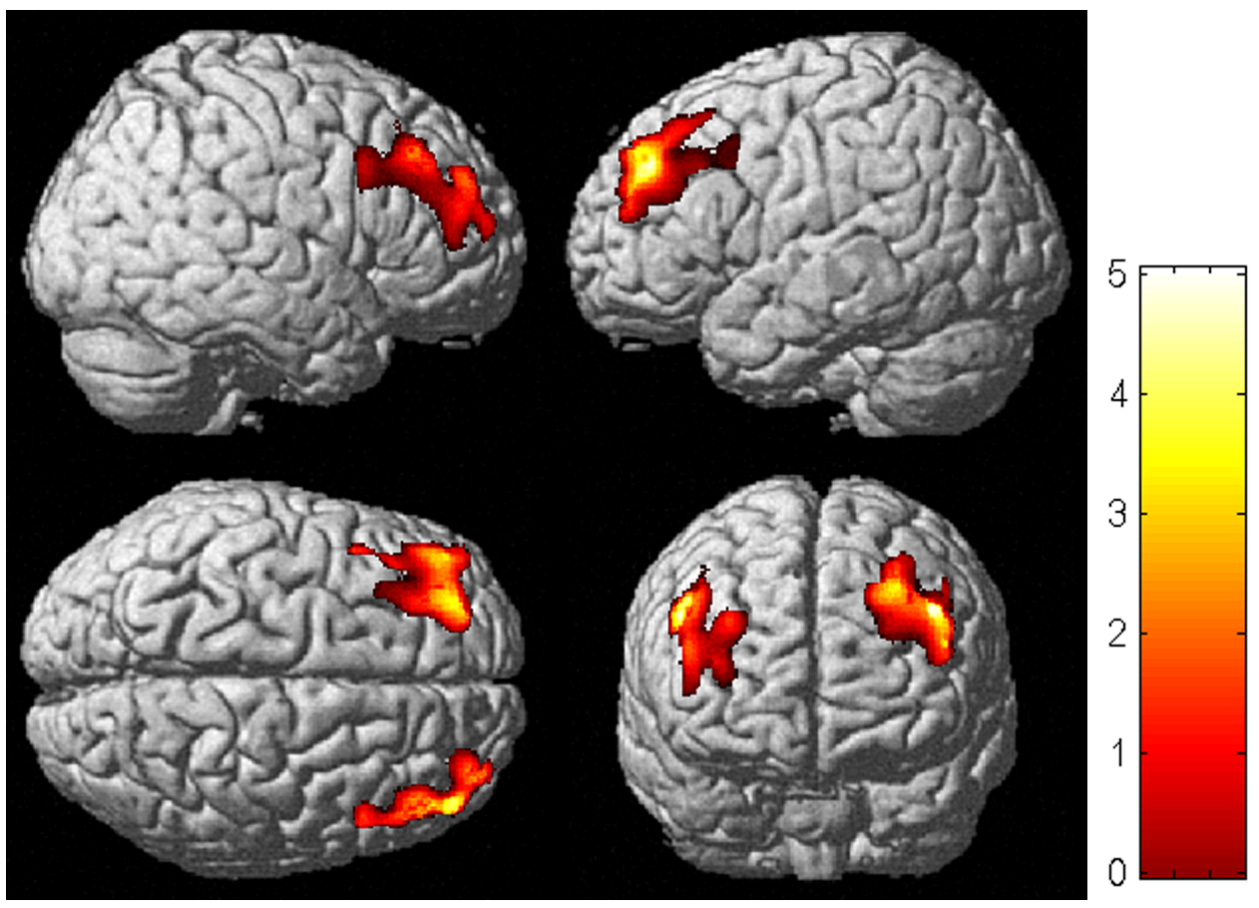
Regional differences between CFS patients and controls. Areas with significantly reduced gray-matter densities in the CFS patients were located at bilateral prefrontal areas, which were surface rendered onto the high-resolution MRI. The colored bar indicates the t-values.
